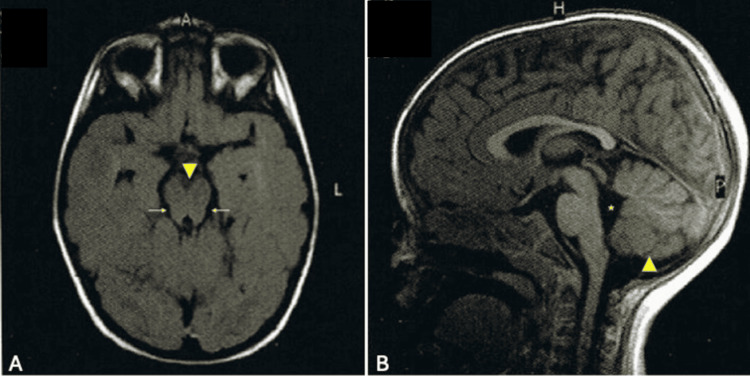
Brain MRI performed at 18 months of age (A) Axial T1-weighted image demonstrates a deepened interpeduncular fossa (arrowhead) and a “molar tooth sign” with slightly elongated, thickened, and horizontally oriented superior cerebellar peduncles (arrows), although not in its most typical form in Joubert syndrome. (B) Sagittal T1-weighted image evidences hypoplasia of the cerebellar vermis, with poor delineation of its lower portion (arrowhead), and enlargement of the fourth ventricle (asterisk).
